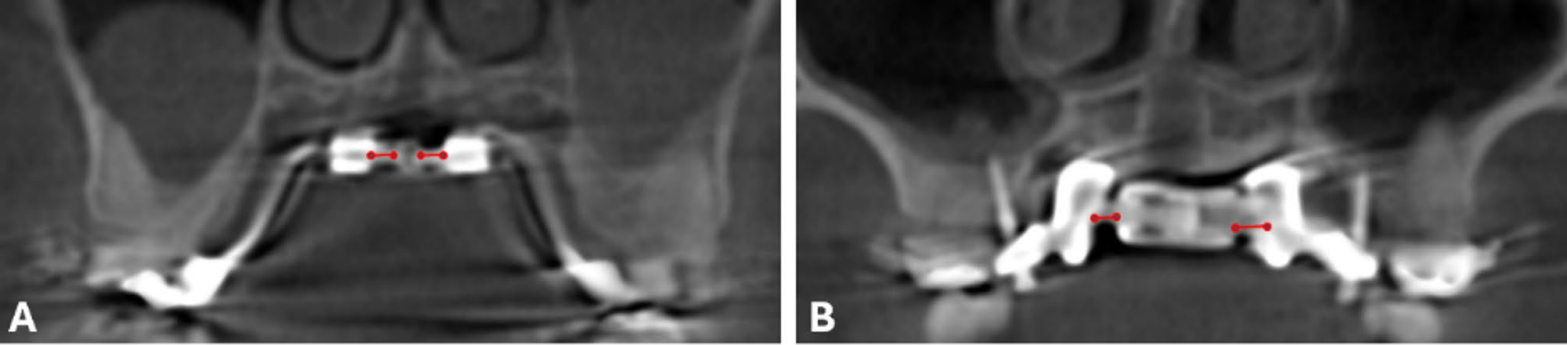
MARPE jackscrew expansion measurements on CBCT at T2. A conventional MARPE; B Custom MARPE
